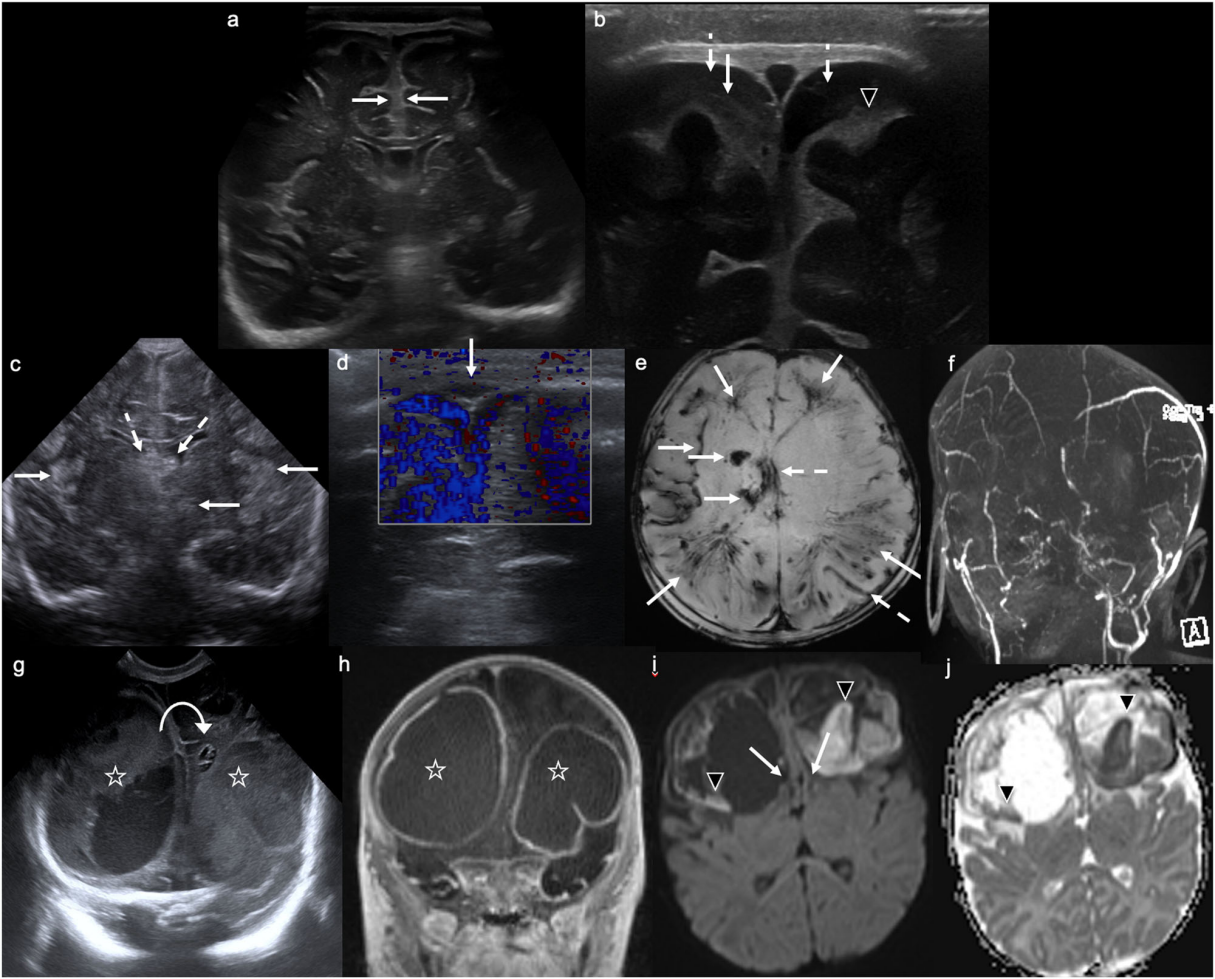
Bacterial meningitis. Ultrasound at 4 months in a drowsy infant with a bulging anterior fontanelle. a Coronal image demonstrates increased echogenicity in the interhemispheric subarachnoid space (solid arrows). b Coronal image with a high-frequency linear probe reveals bilateral hypo-/anechoic parafalcine subdural collections (left > right) (dashed arrows), increased echogenicity of the right parasagittal subarachnoid space (solid arrow), and widening of left frontal sulci (black arrowhead) due to distension of the subarachnoid space by purulent material. Findings are consistent with bacterial meningitis—caused by *Haemophilus influenzae* in this case. Ultrasound at 3 weeks in a neonate presenting with septic shock. c Coronal image demonstrates ill-defined, bilateral (left > right), multifocal cortical, subcortical, and deep grey matter increased echogenicity (solid arrows). There is also echogenic material in the frontal horns of both lateral ventricles, suggesting purulent/infectious debris or intraventricular haemorrhage (dashed arrows). d Coronal colour Doppler image demonstrates absence of flow in the superior sagittal sinus (solid arrow). e Axial SWI reveals corresponding bilateral susceptibility artefact, consistent with haemorrhage, involving the right insular cortex, bilateral frontal and parietal subcortical white matter, and the right corpus striatum (solid arrows); linear susceptibility artefact represents cortical and medullary vein thrombosis (dashed arrows). f Coronal MRV demonstrates a lack of signal in most of the dural venous sinuses (superior sagittal, transverse, and sigmoid), consistent with extensive dural venous sinus thrombosis. Findings are consistent with meningoencephalitis—due to Group B *Streptococcus* sepsis in this case, with extensive secondary haemorrhagic venous infarction. Ultrasound at 28 days in a term neonate presenting with desaturations and cyanosis. g Coronal image demonstrates large, bilateral ovoid/“box”-shaped frontal lobe masses, right > left (*), with mixed internal echogenicity, consistent with abscesses. Minor leftward midline shift is present (curved arrow). h Coronal post-contrast T1WI details thick rim enhancement of bilateral frontal lobe abscesses (*). i Axial trace DWI and j ADC map show globular restricted diffusion within the abscesses, indicating pus (black arrowheads); note the effacement of the frontal horns of both lateral ventricles due to mass effect (solid arrows). Findings are consistent with bilateral frontal lobe abscesses, due to *Citrobacter* infection in this case
